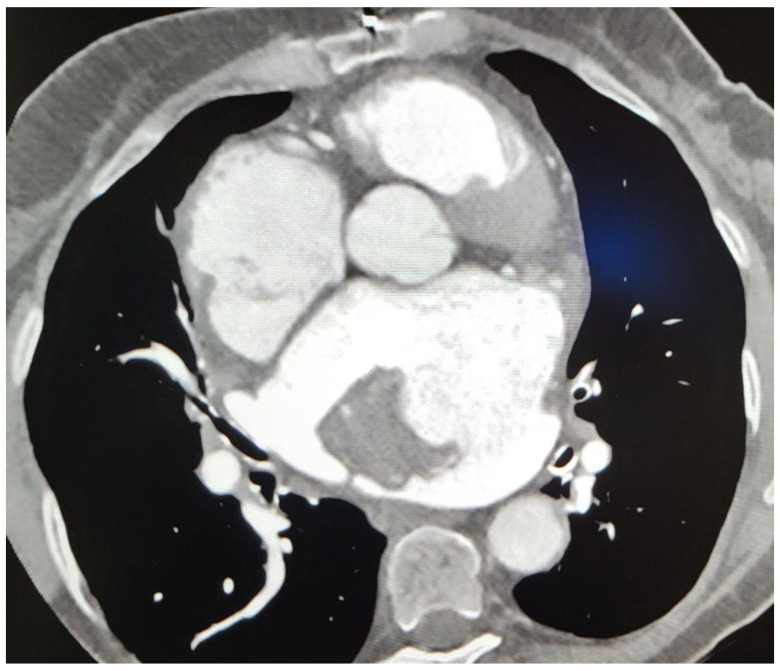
Computerized tomography (CT). Sharply limited heterodense change with a diameter of 53 × 46 × 37 mm and a wide base attached to the wall of the atrium.
